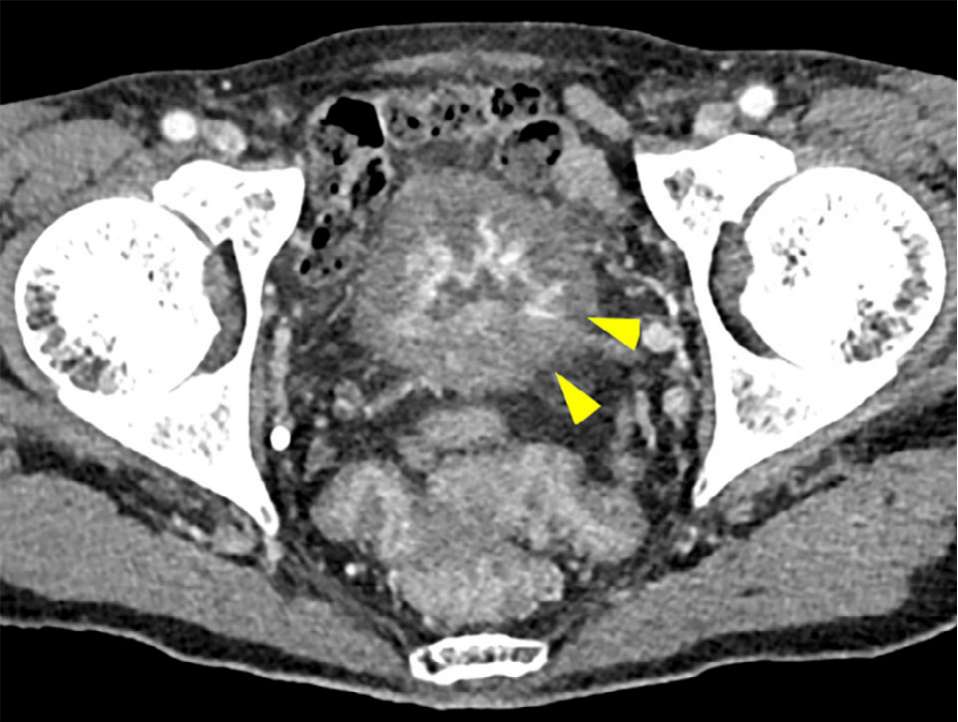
Pelvic imaging with contrast‐enhanced CT. The bladder wall is thickened with contrast enhancement of the mucosa (arrowhead).
